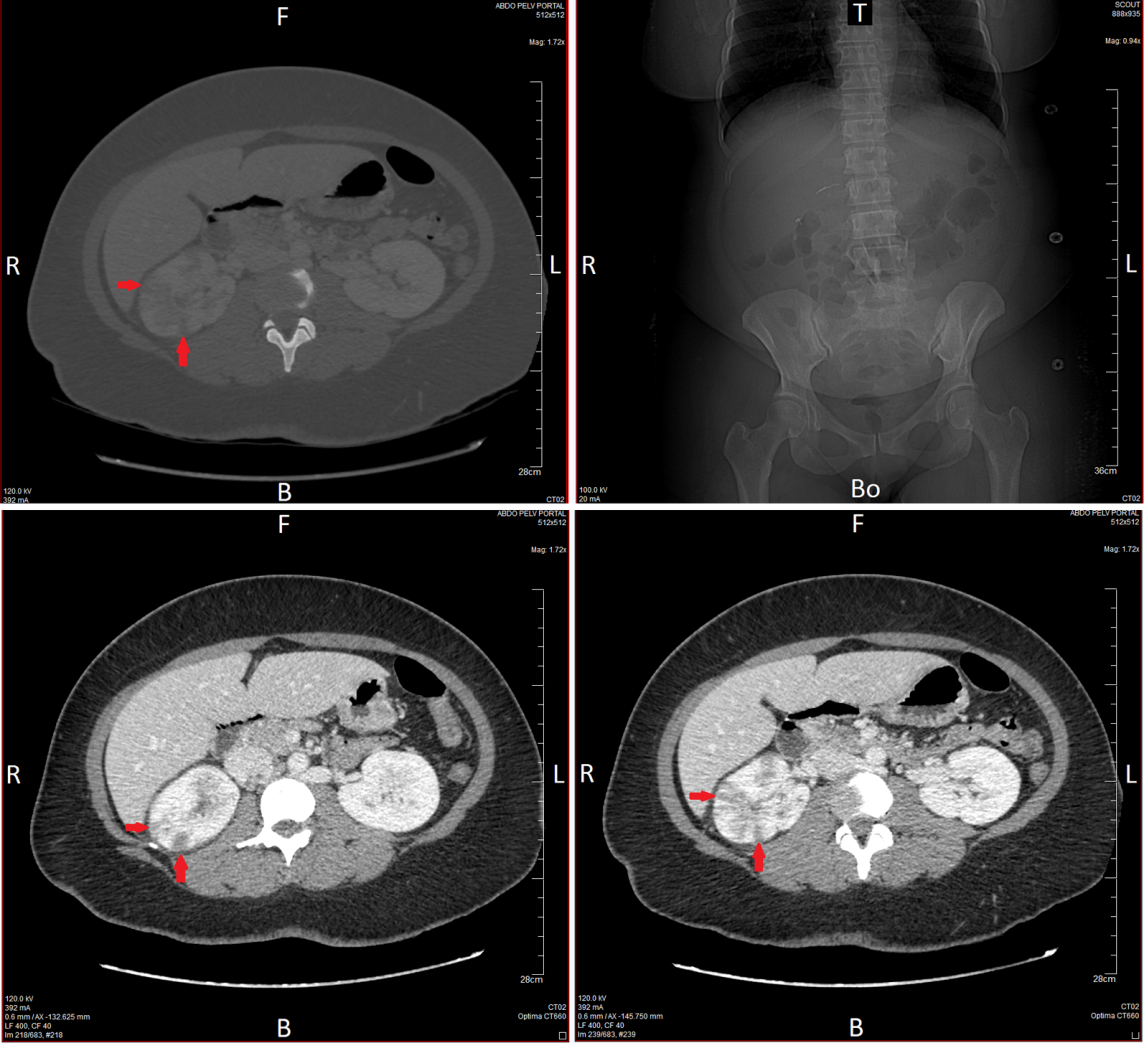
Multiple foci of nephritis of the upper pole of the right kidney. Axial CT slice through the abdomen from CT scans without injection of intravenous contrast (photo 1), CT Scout view (photo 2), and with injection of intravenous contrast (photos 3 and 4). B, back; Bo, bottom; F, front; L, left; R, right; T, top. CT scan found multiple triangular hypodensities (indicated by the red arrows) of the right superior polar renal cortex in favor of foci of nephritis. Absence of contrast of the right pyelic walls. There was no dilatation of the right and left pyelocaliceal cavities. There was no parenchymal abnormality of the left renal pelvis. The bladder was in low replenishment. There was no evidence of lithiasis in the urinary excretory tract. There was no abnormality in the hepatic, splenic, pancreatic, or adrenal compartments. No intraperitoneal or retroperitoneal effusion was observed. There was a good permeability of the vascular axes. Conclusion: multiple foci of nephritis of the upper pole of the right kidney; no dilatation of the pyelocaliceal cavities or obstruction of the urinary excretory tract
